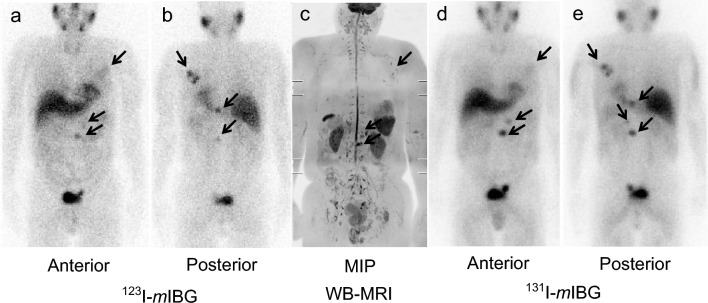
A case of multiple bone metastases (patient No. 6). A male patient with multiple bone metastases of pheochromocytoma (left scapula, thoracic, and lumbar vertebra, as indicated by black arrows). ^123/131^I-*m*IBG images detected more lesions compared with WB-MRI. Lumbar vertebral metastases were detected clearly by ^131^I-*m*IBG images. (a,b) ^123^I-*m*IBG scintigrams (anterior and posterior). (c) The MIP of WB-MRI. (d,e) Post-therapy ^131^I-*m*IBG scintigram (anterior and posterior). *mIBG meta*-iodo-benzylguanidine, *MIP* maximum intensity projection, *WB-MRI* whole-body magnetic resonance image.
